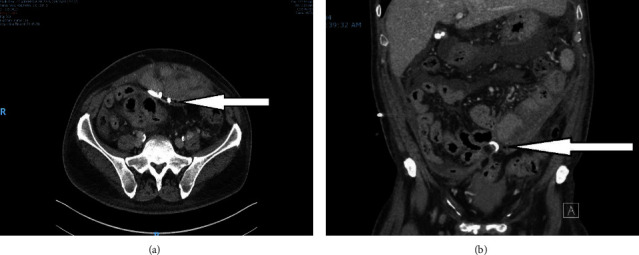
(a) Computed tomography (CT) abdomen/pelvis cross sectional view showing near complete resolution of the previously large intraabdominal abscess with small amount of air visible along the track of the drain catheter (solid white arrow). (b) Computed tomography (CT) abdomen/pelvis coronal view showing near complete resolution of the previously large intraabdominal abscess with air visible along the track of the drain catheter (solid white arrow).
